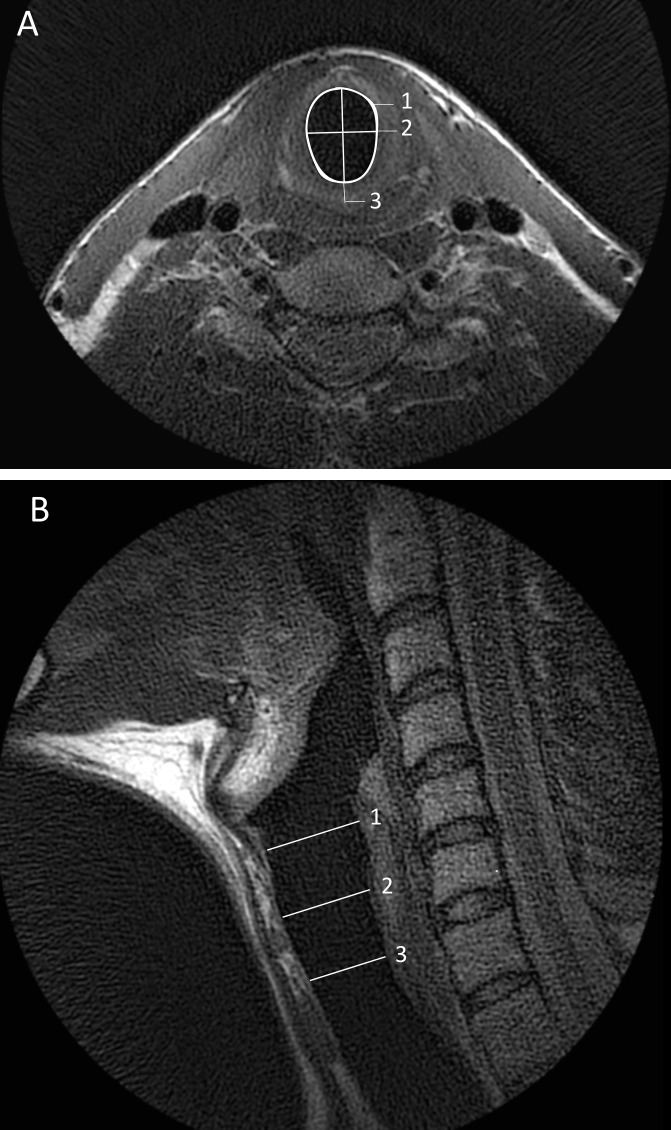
Example of MRI analysis on a healthy volunteer (A) axial T2-weighted image showing the measurement of the lumen area (1), Left right (2) and transversal diameter (3) at the level of the Cricoid, (B) sagittal T2-weighted image showing the location of the cricoid (1), the proximal trachea (2) and the most caudal slice (3).
